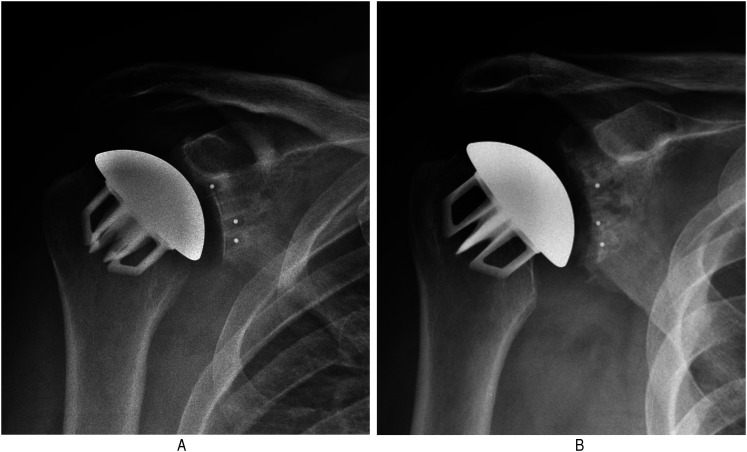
(a) Three-month postoperative anteroposterior (AP) radiograph and (b)
